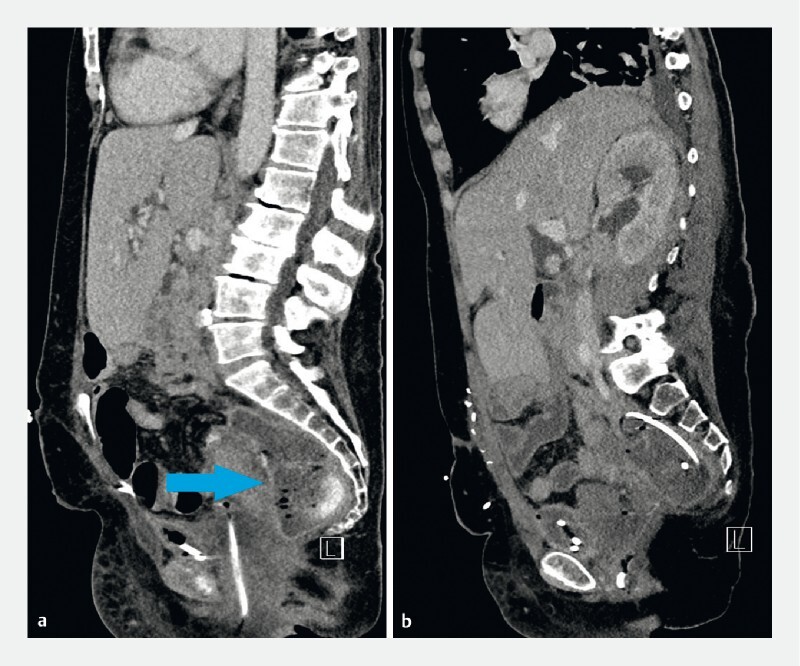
Contrast-enhanced computed tomography image of the abdomen showing:
